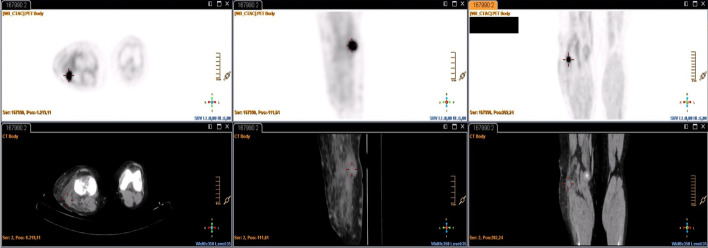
43-year-old female with low-grade malignant peripheral nerve sheath Tumor. PET CT showed a solid nodule in the right lower limb with homogeneous uptake of ^18^F-FDG
